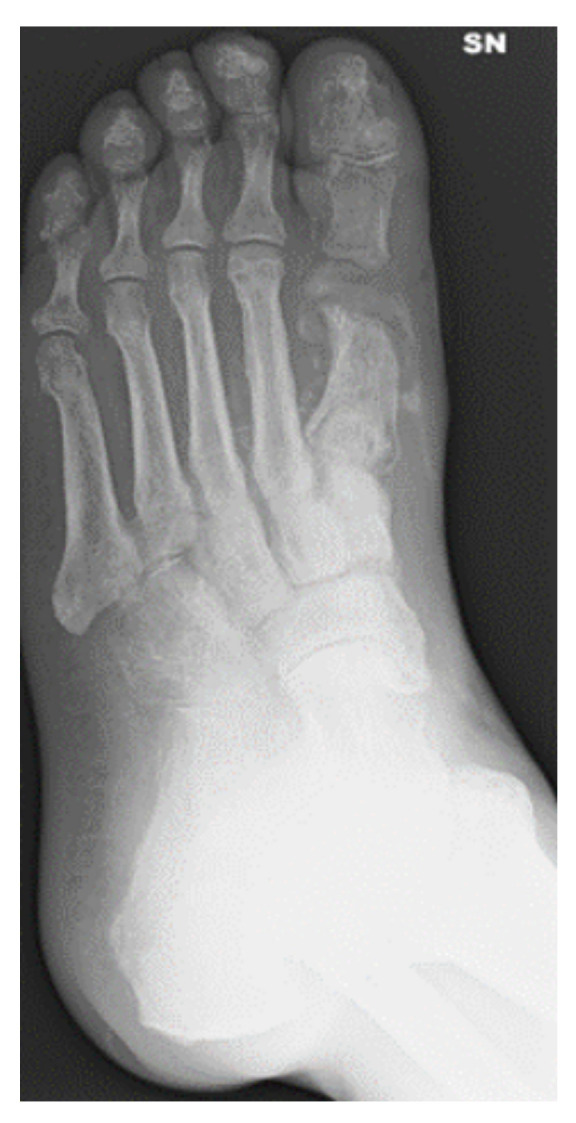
Final radiographic outcome at 4 months.
